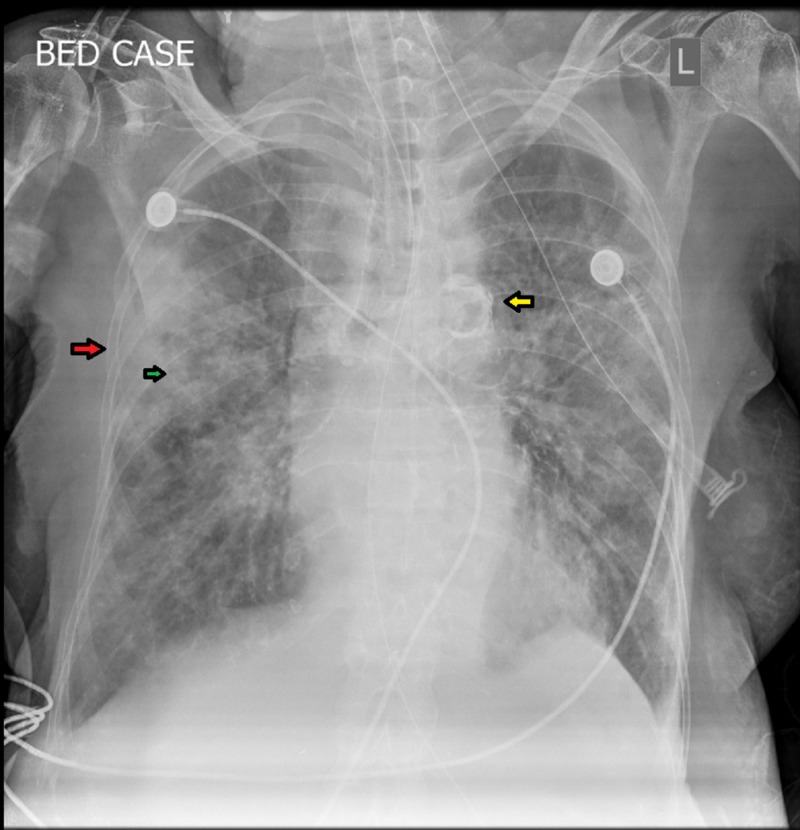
Chest X-ray depicting pathological changes of pneumonia in our patient L: Left-sided orientation of the patient Bed case: Bedside chest X-ray Widespread opacification of the respective right and left lung fields can be observed. The right upper and middle lung zone opacities have fused to impart a wedge-shaped area of opacification (red arrow). Within this wedge-shaped area, dark air-filled bronchi are being made visible by the surrounding opacification of alveoli (air bronchogram, green arrow). Physiological calcification of the aortic knuckle can also be noted (yellow arrow).
